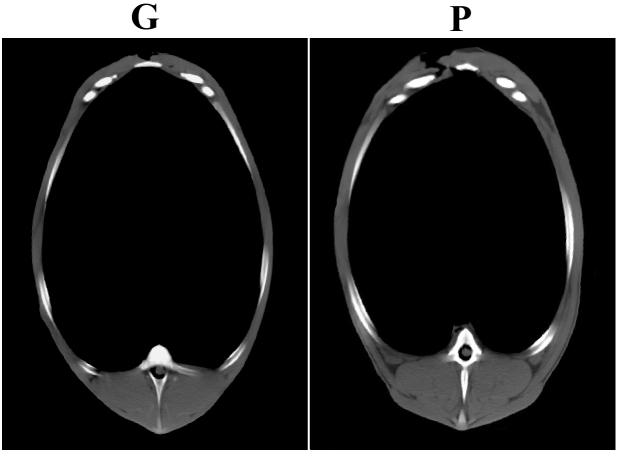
Cross sectional CT scan at identical anatomical location (at the joint
